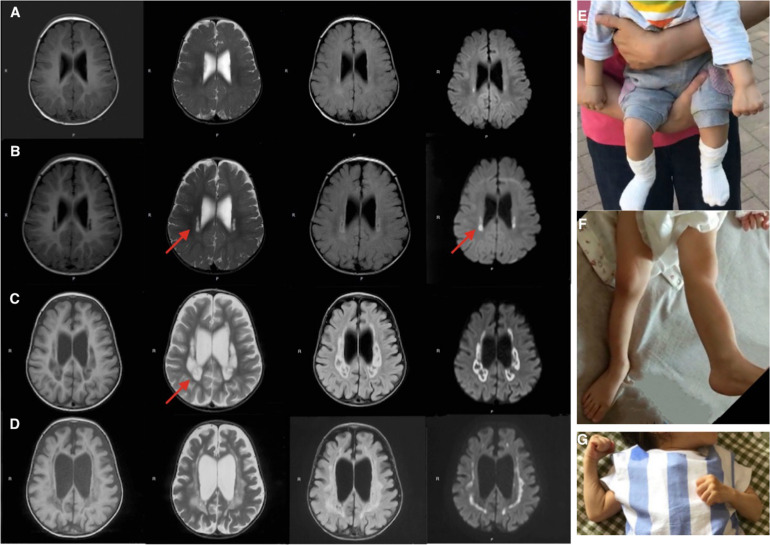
Brain MRI (T1-weighed) showing progressive brain atrophy. MRIs of the patient taken at (A) 9, (B) 11, (C) 16, and (D) 21 months of age. Note the high T2 signal in the periventricular regions on restriction diffusion weighed images (B,C). (E,F) Progressive muscular hypertonia at 11 and 16 months old. (G) Partially controlled hypertonia after baclofen treatment at 2 years old.
